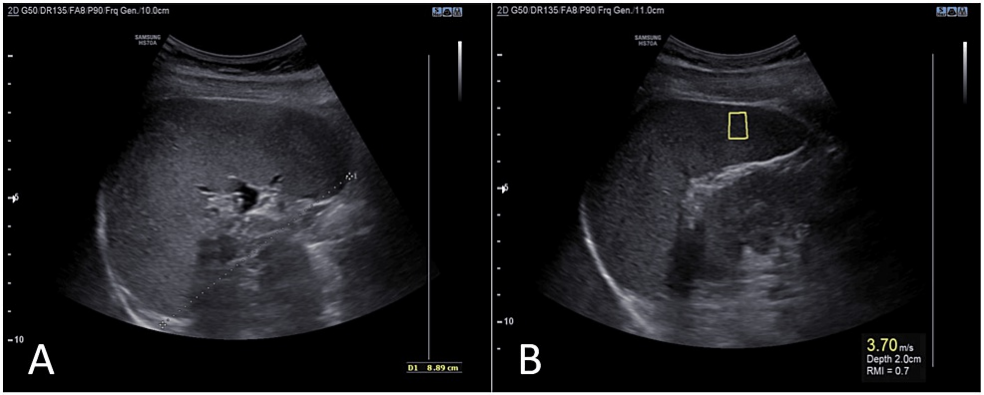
B-mode ultrasound image showing longitudinal spleen diameter measurement and pSWE image showing the region of interest (ROI) B-mode ultrasound image showing the longitudinal spleen measurement (dotted line) (A) and point shear wave elastography depicting the ROI (yellow box) (B).
